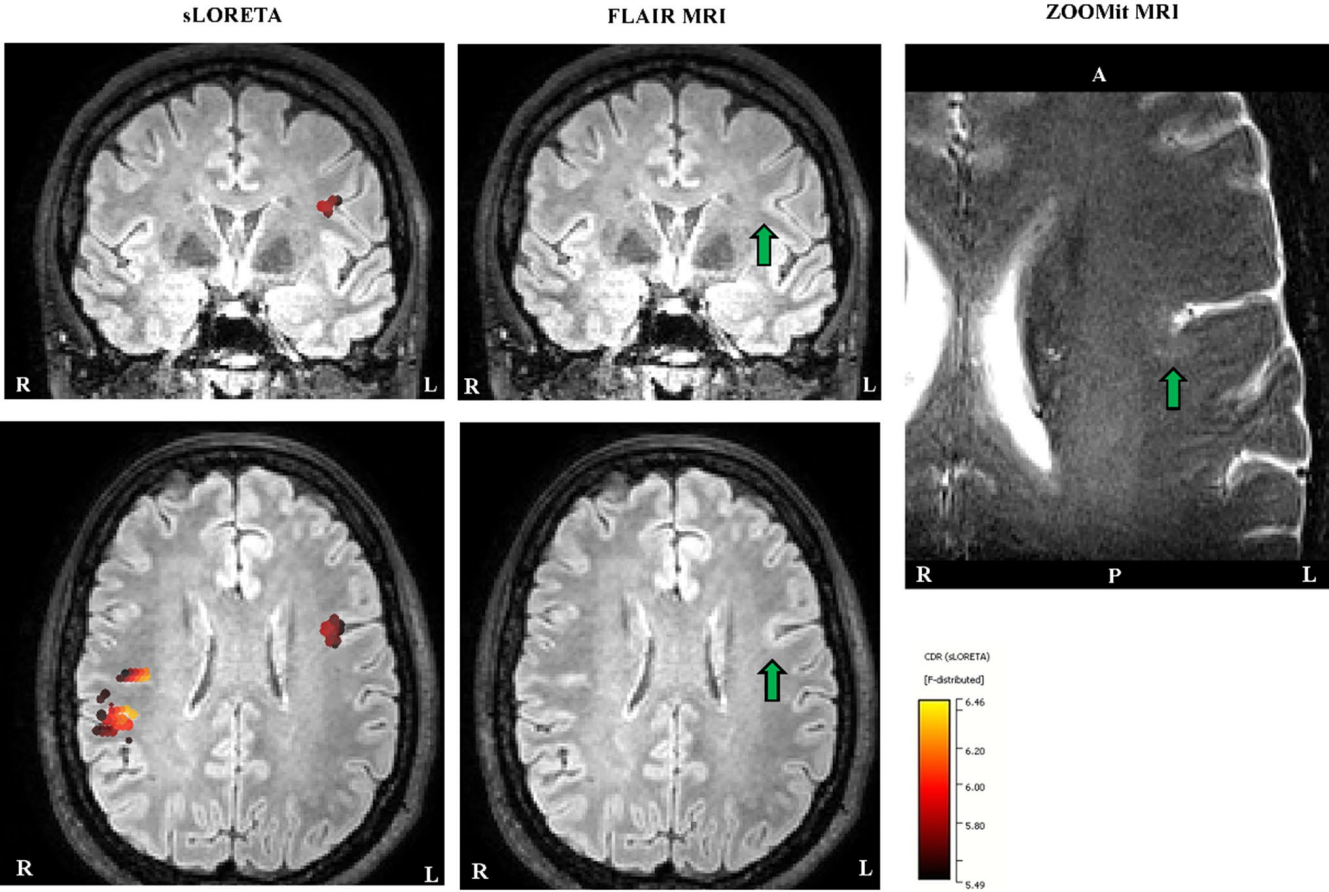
EMEG source localizations at −23 ms, slices selected according to the left hemispheric activity. In the left column, the sLORETA results registered to the FLAIR MRI are shown (only results obtained with a threshold of 85% for the maximum F-value are shown). In the middle column, the FLAIR image without localizations is shown, in order to enable the identification of the FCDs (pointed to by *green arrows*). The right column shows the ZOOMit MRI for the localization cluster (detected with sLORETA) at the left fronto-central region with the *green arrow* indicating the FCD. *White letters* on the MRIs show the directions (*L* left, *R* right, *A* anterior, *P* posterior). (Color figure online)
